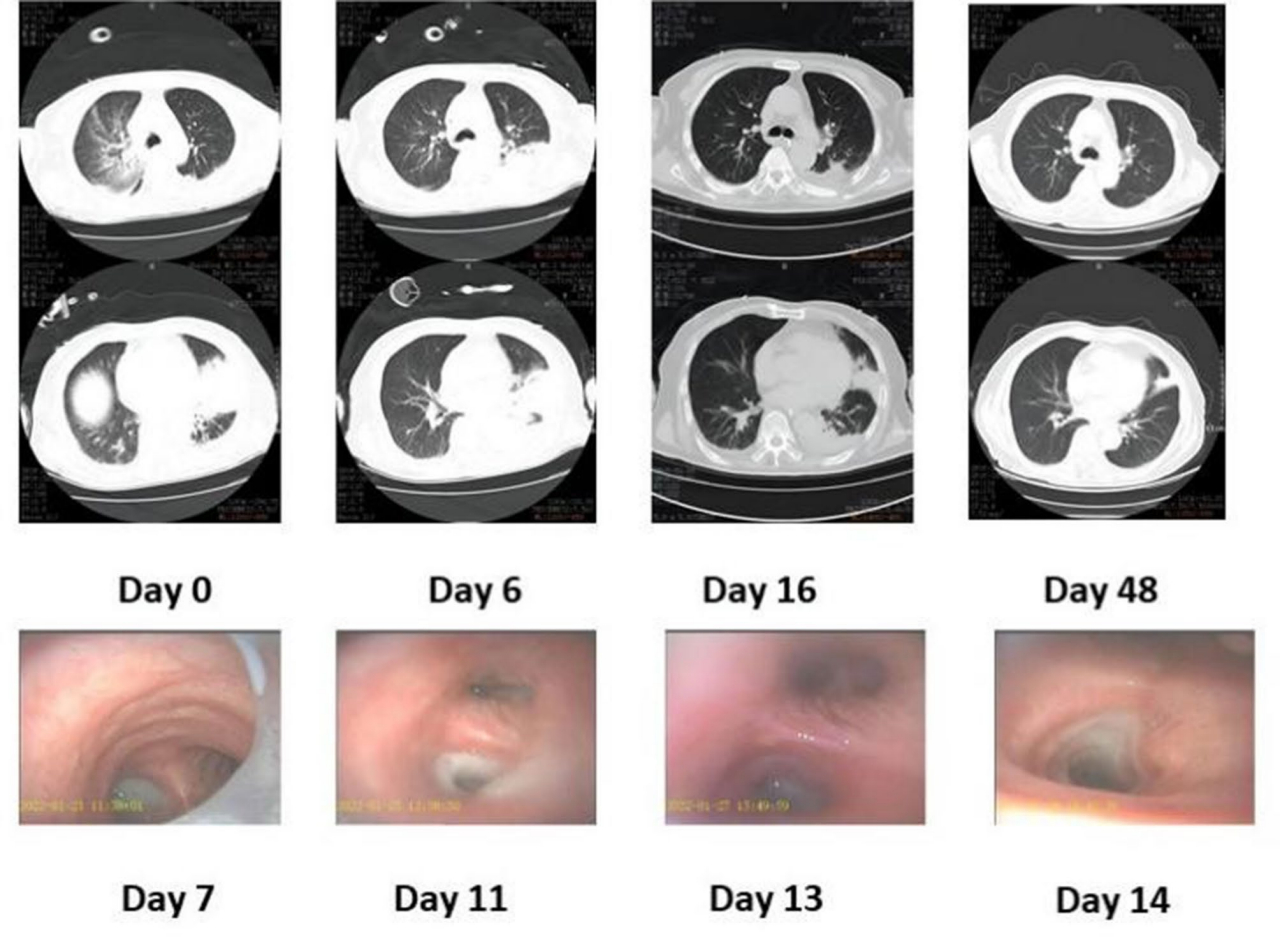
Results of fiberoptic bronchoscopy and chest CT. Fiberoptic bronchoscopy showed that trachea cannula and massive yellow purulent sputum in the left main bronchus on day 7. Reexamination on days 11, 13, and 14 showed a gradual decrease of sputum. The follow-up chest CT on the 6th day revealed an increased extent of pneumonia. After a 10-day course of doxycycline treatment and multiple bedside fiberoptic bronchoscopies with sputum aspiration, significant improvement in pneumonia was observed on the 16th, 22nd, and 48th days
